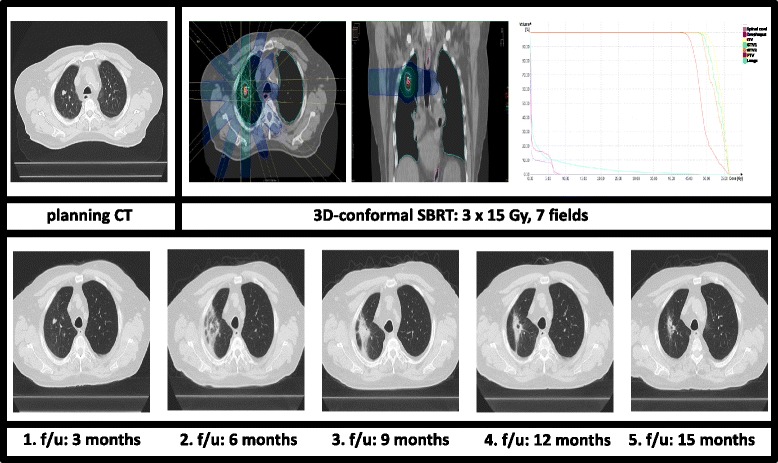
Treatment planning with dose-volume-histogram (PTV and OARs) and follow-up CT-scans for a peripheral pulmonary tumor. Radiation associated imaging changes: increasing perilesional, ground glass opacity leading to fibrosis
